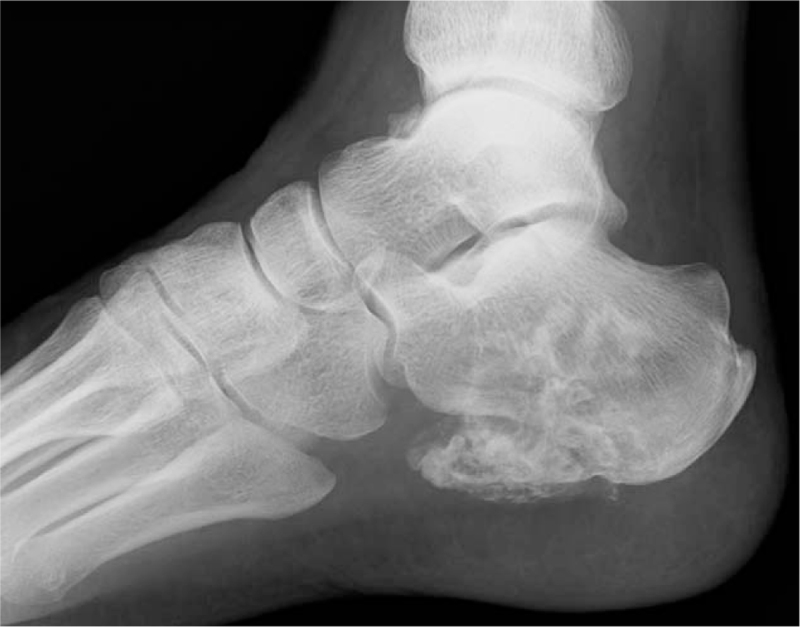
A lateral radiograph demonstrates radiolucency with an extraosseous mineralized mass in the calcaneal body.
